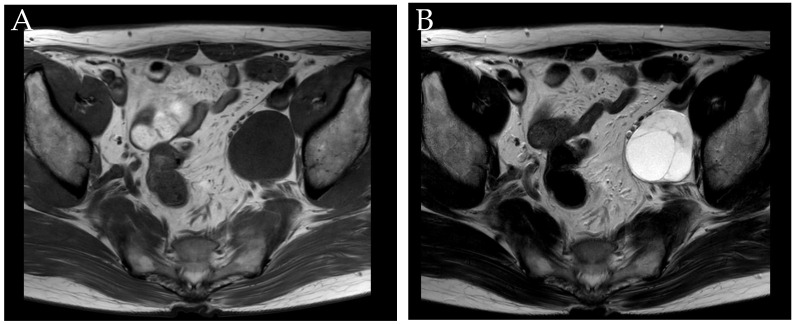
(A) A T1-weighted magnetic resonance image shows a homogenous low-signal-intensity mass, while (B) the T2-weighted MR image reveals several cysts with septa within the tumor.
